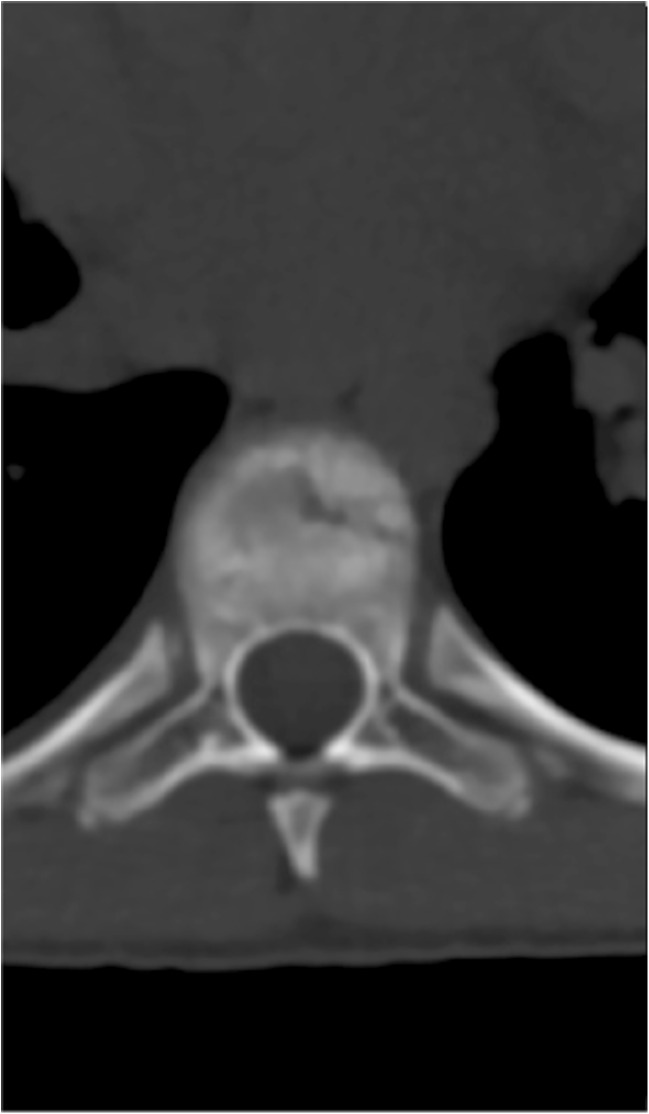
CT scan of fractured VB (axial)
